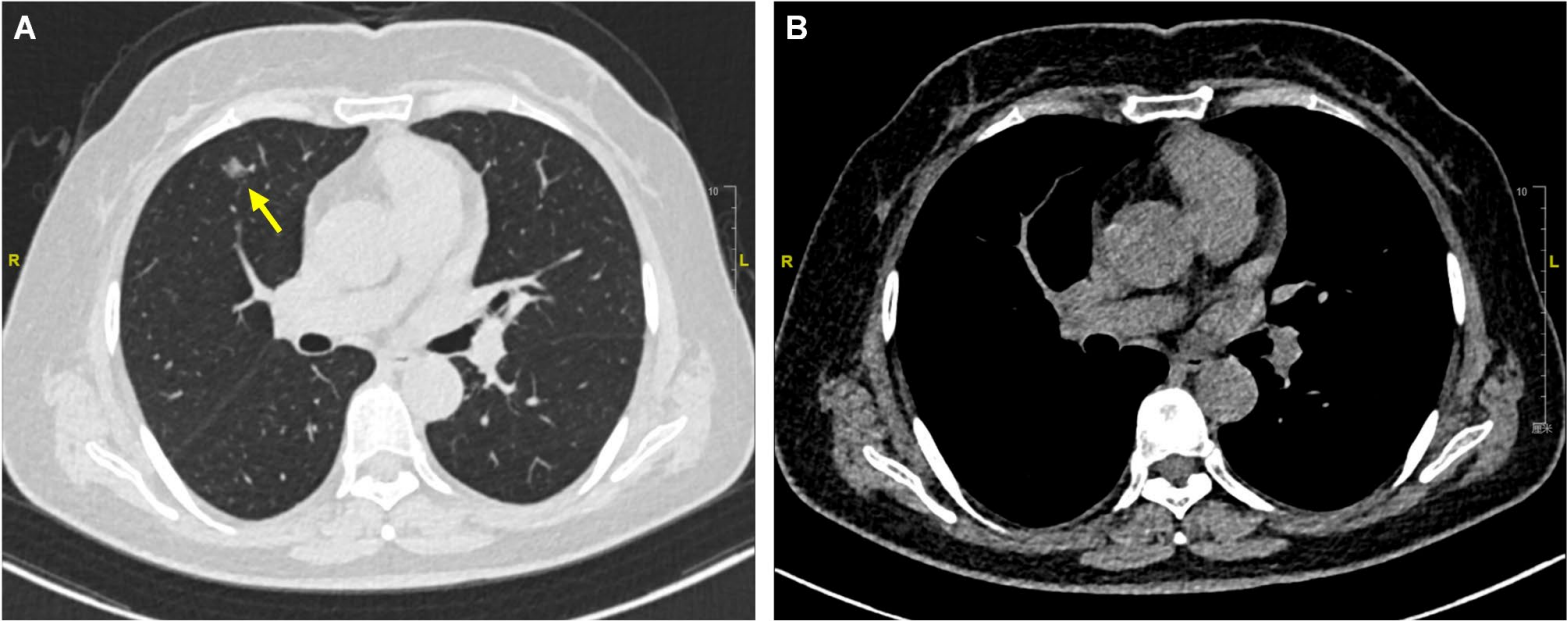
A chest CT revealed a GGO (yellow arrow) in the anterior segment of the right upper lobe (Subfigure A). The size of the GGO was approximately 11*9 mm in the lung window, and no visible solid component was observed in the mediastinal window (Subfigure B)
